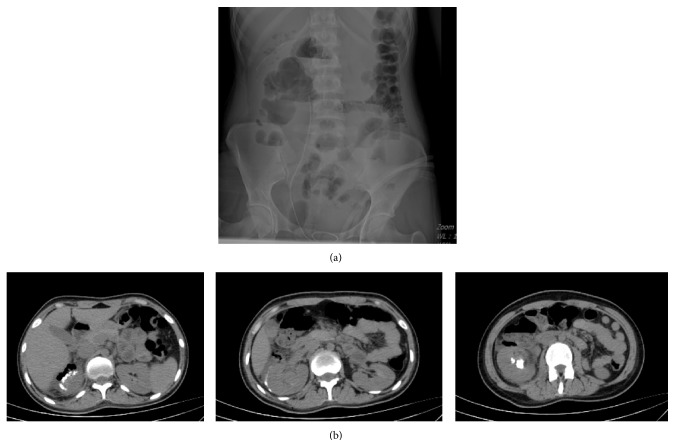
(a) Kidney, ureter, and bladder X-ray in postoperative day 1. (b) Perirenal urinoma, perirenal and infrahepatic stone fragments, and lower calyceal residual stone fragments were seen on computed tomography in postoperative day 1.
